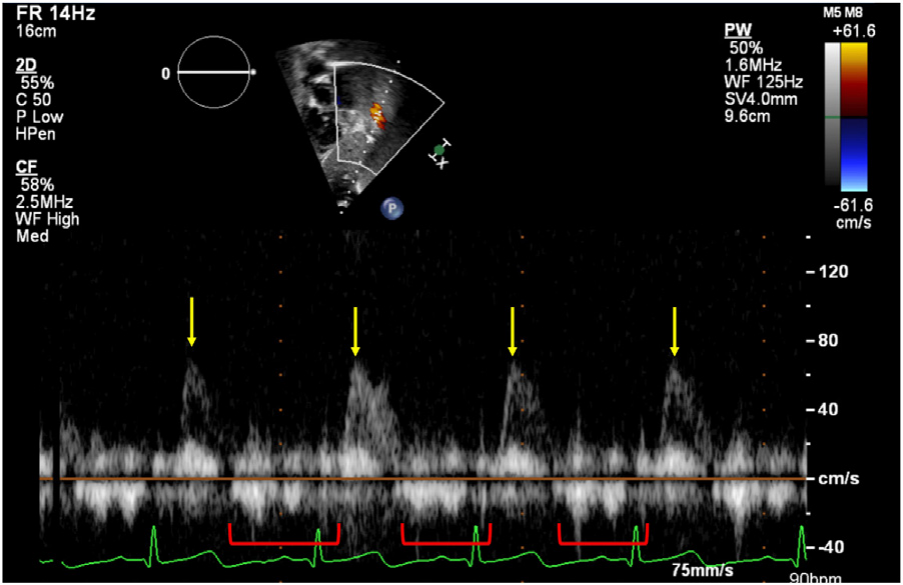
Two-dimensional TTE subcostal sagittal view with color flow–guided pulsed-wave Doppler spectrum demonstrates a pulsatile abdominal aortic Doppler *(arrows)* pattern without a diastolic runoff pattern *(red bracket)* at this angle of interrogation.
